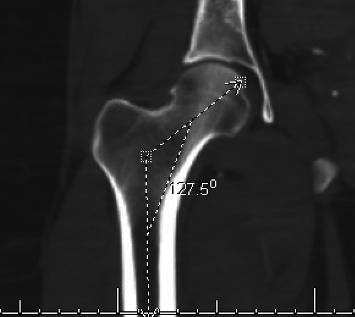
Measurement of CCD angle via CT image in EMR/PACS, with 127.5 degrees representing the CCD angle.
